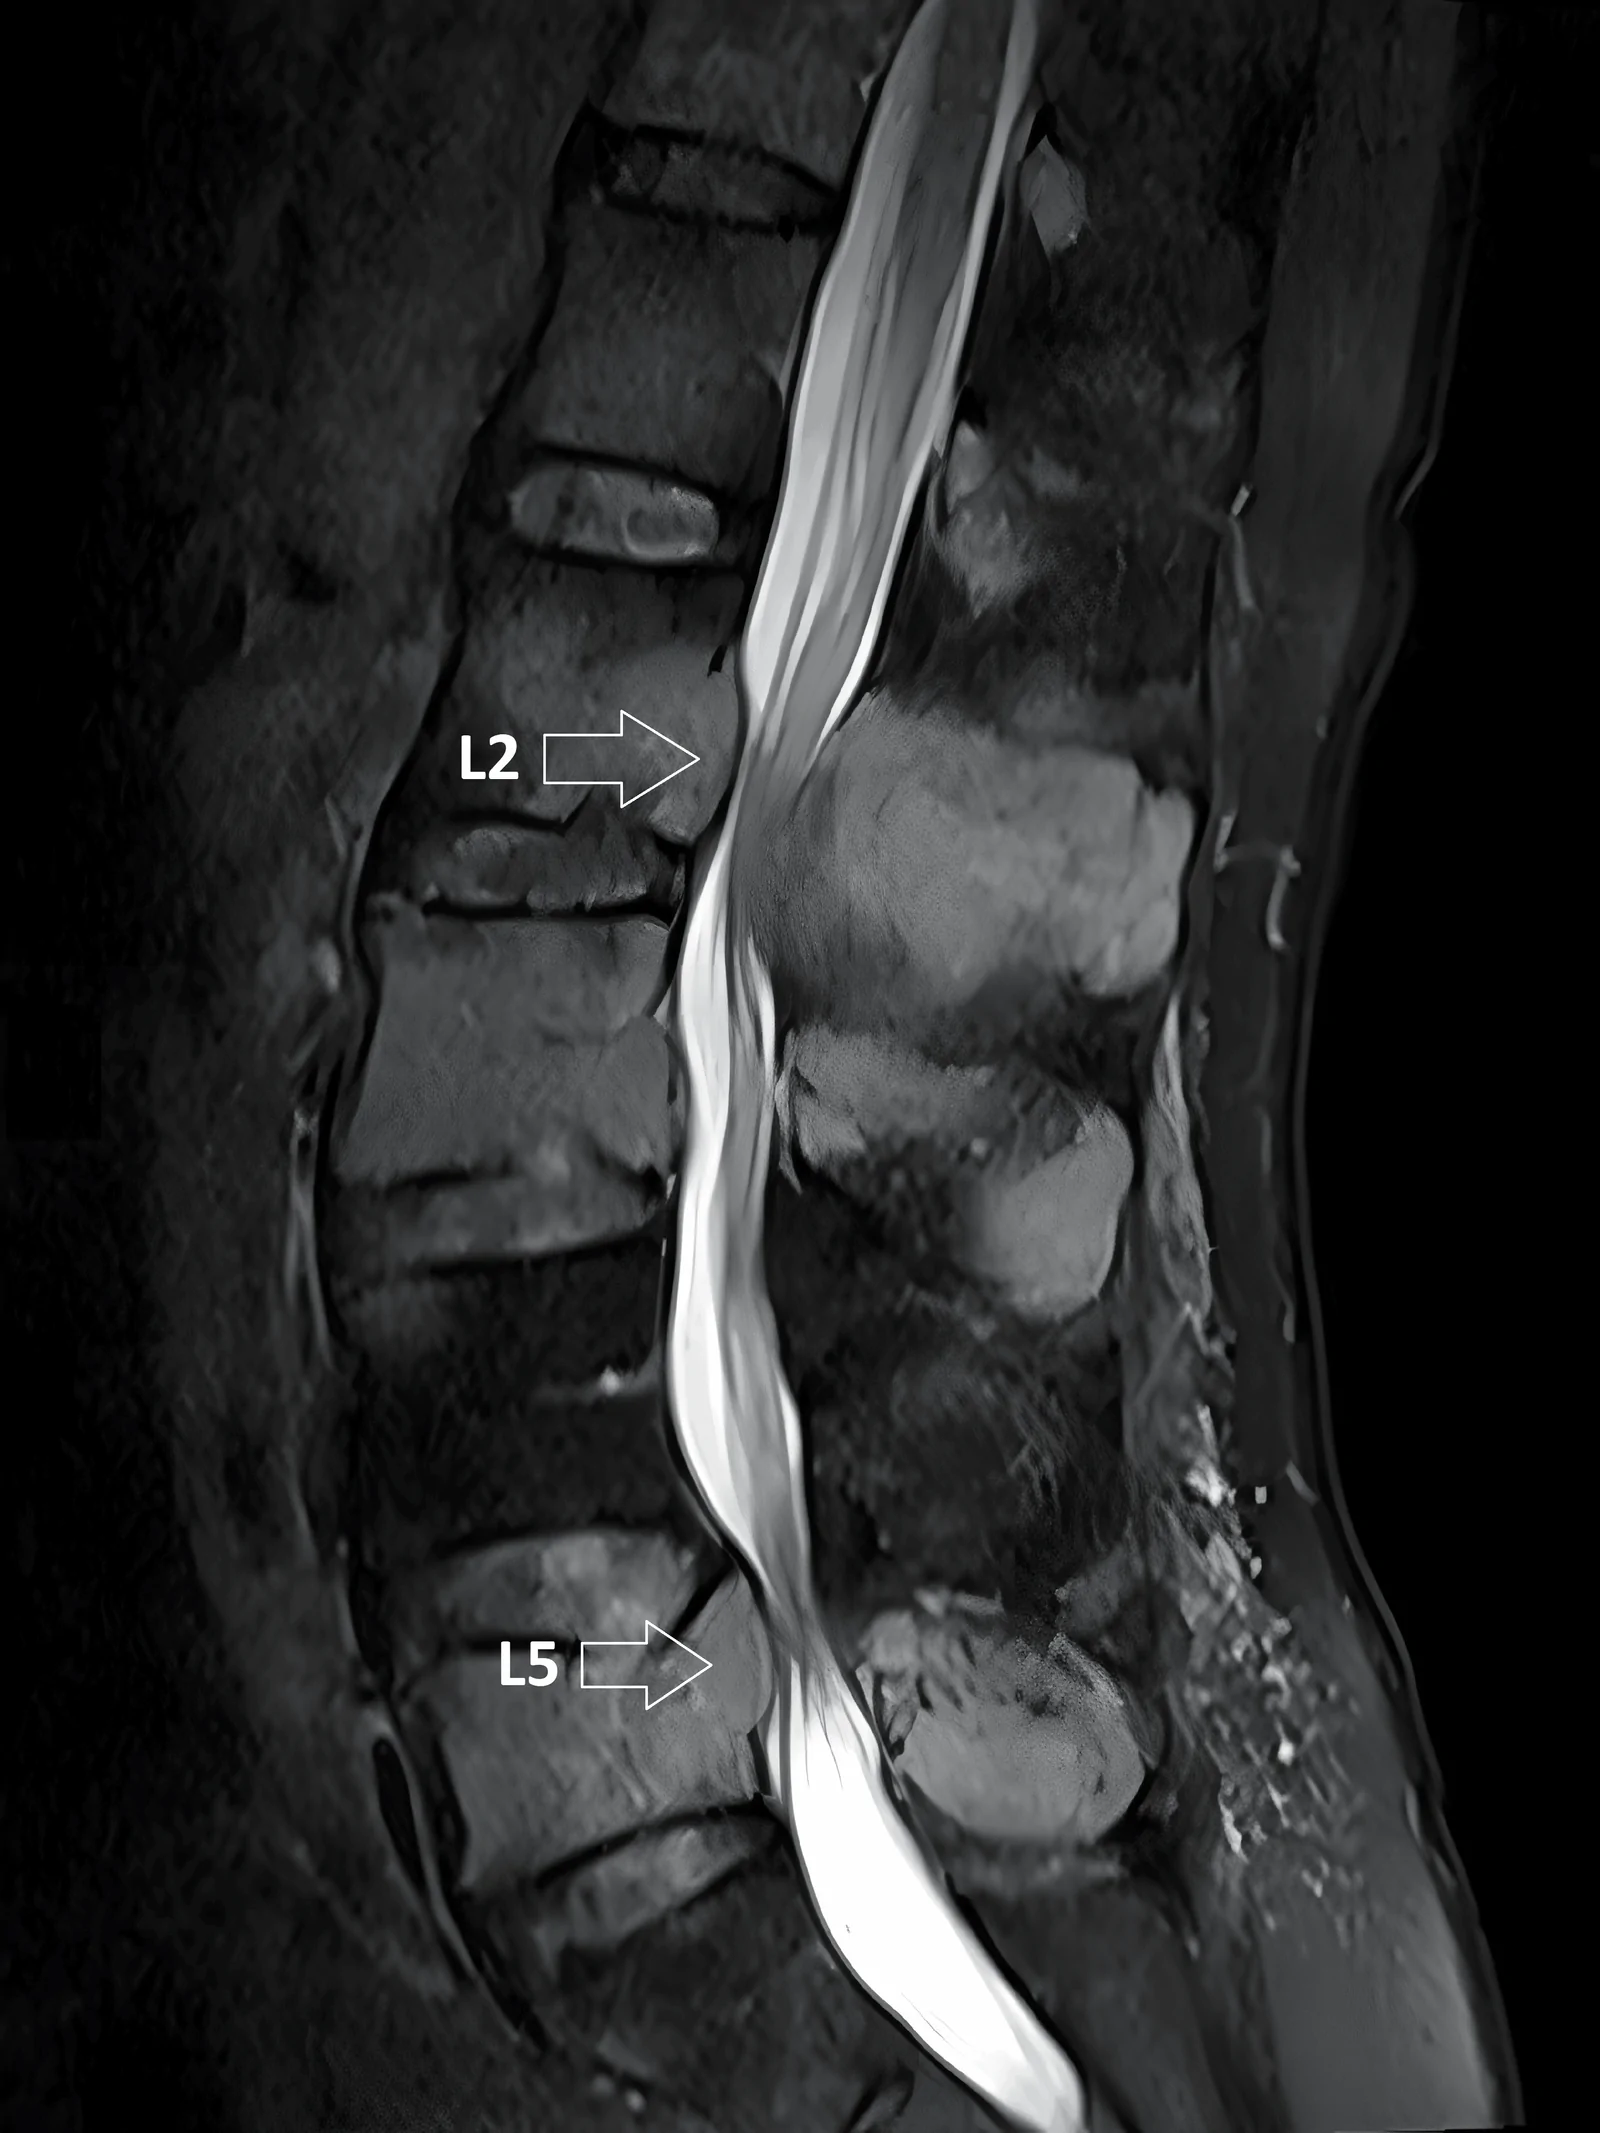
MRI of the lumbar spine. At L2 and L5 levels, severe stenosis is seen in relation to the retropulsion and expansion effect of the bone metastasis (white arrows).
